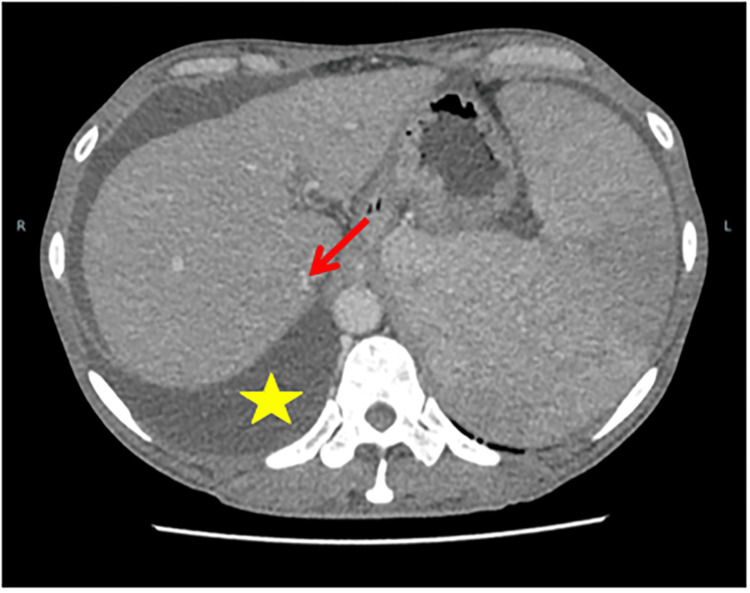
Abdominal CT: The liver was enlarged in size with splenomegaly, the hepatic segment of the inferior vena cava was obviously compressed (shown by the red arrow), and speckled enhancement was seen near the porta hepatis. A large amount of ascites was seen (yellow pentagram).
